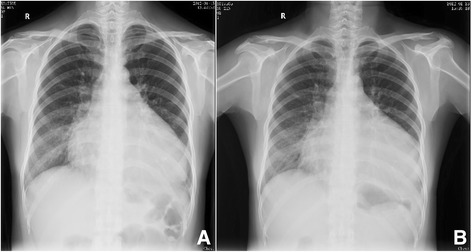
Chest x-ray of pneumonia (a) obtained on January 15, 2012, and (b) x-ray from January 23, 2012
